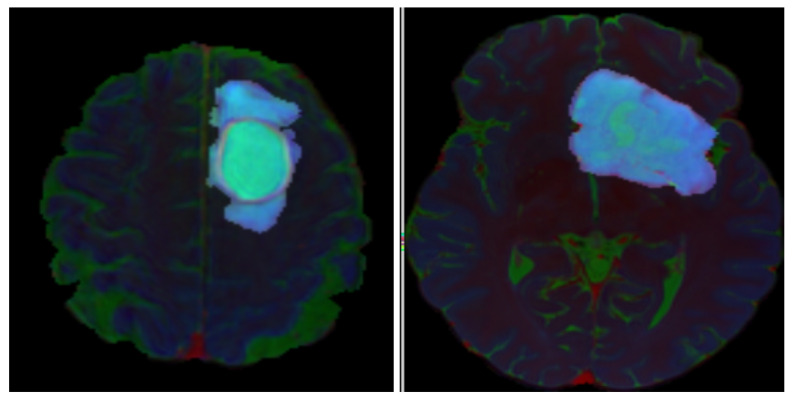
Two example RGB image slices that belong to an IDH-wildtype. (left) and an IDH-mutant (right) glioma from the test dataset of Naïve Soft Filtering. R: FLAIR, G: T1c, B: T2w.
